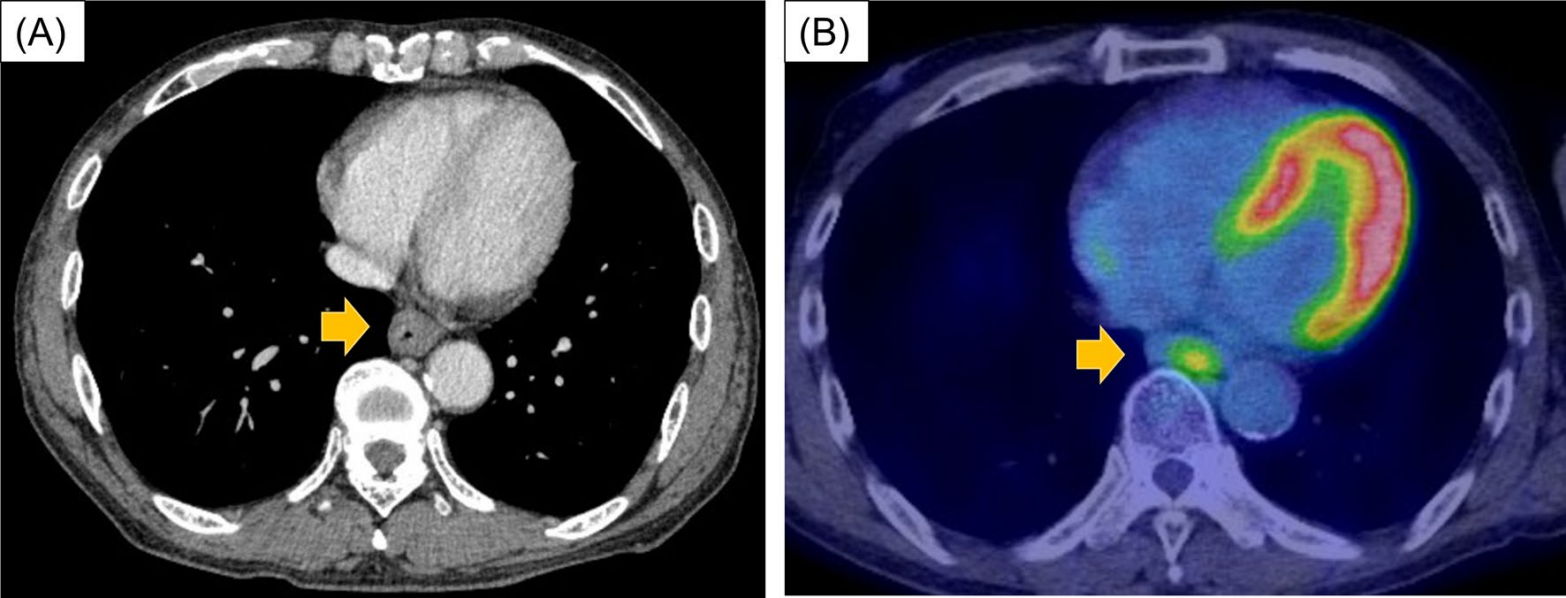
CT showed the tumor as thickness of esophageal wall (A). PET showed fluorodeoxyglucose concentration in the esophageal tumor (B)
